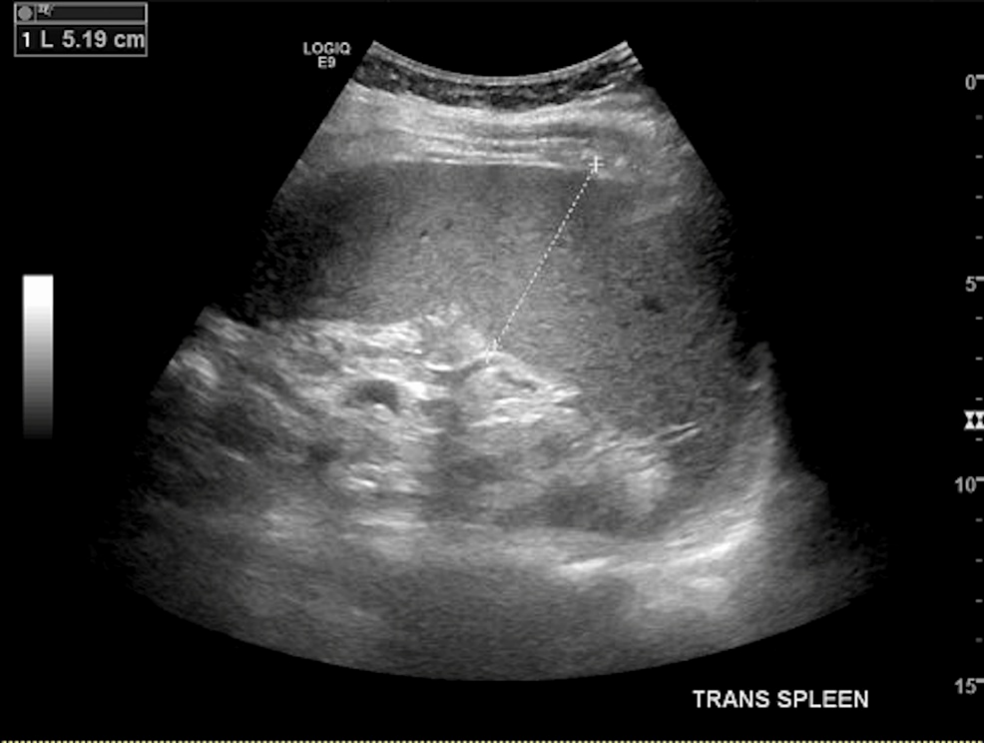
Transverse ultrasound of spleen
